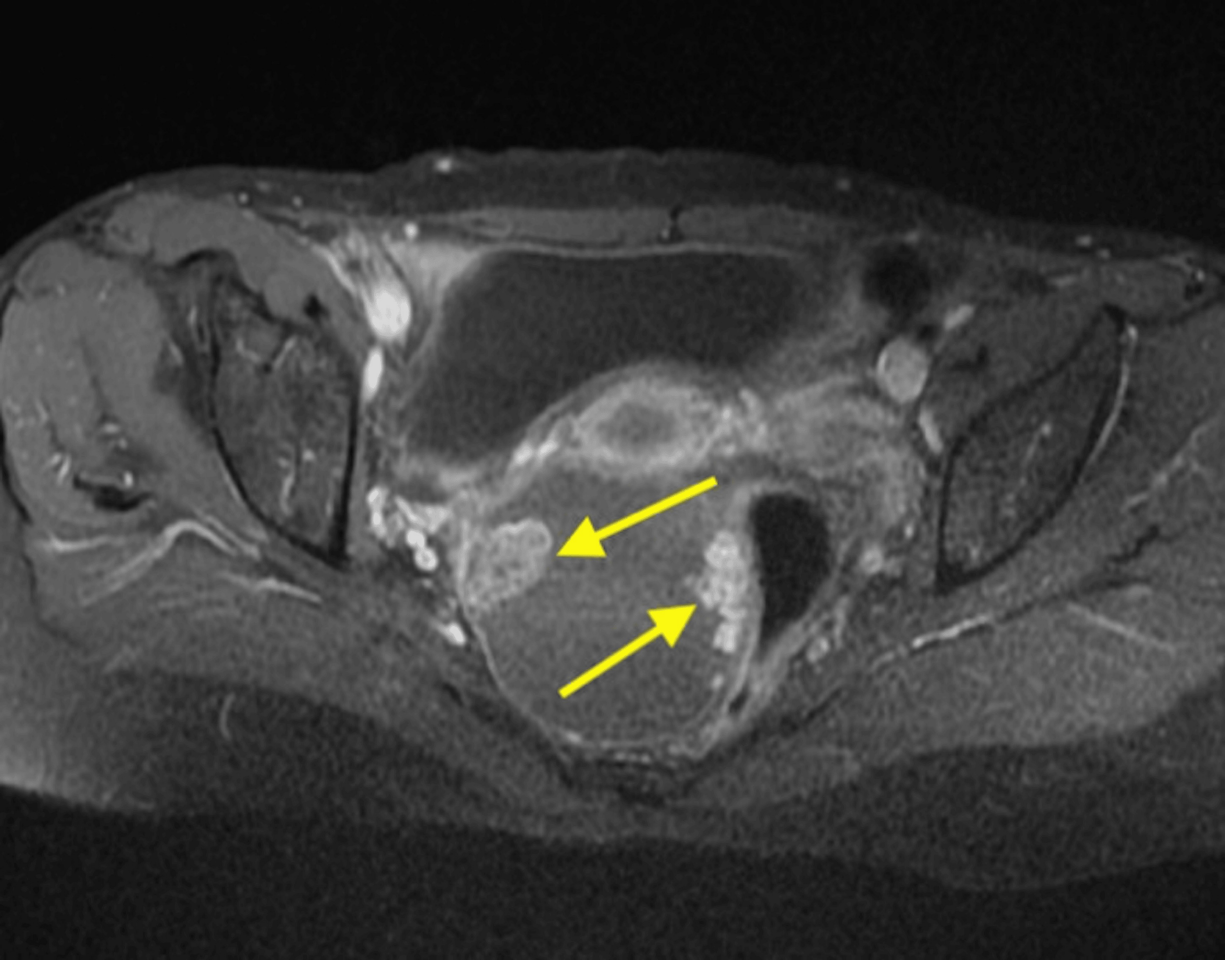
The post-contrast MRI image The image shows the solid pelvic-abdominal lesion. MRI = Magnetic Resonance Imaging
